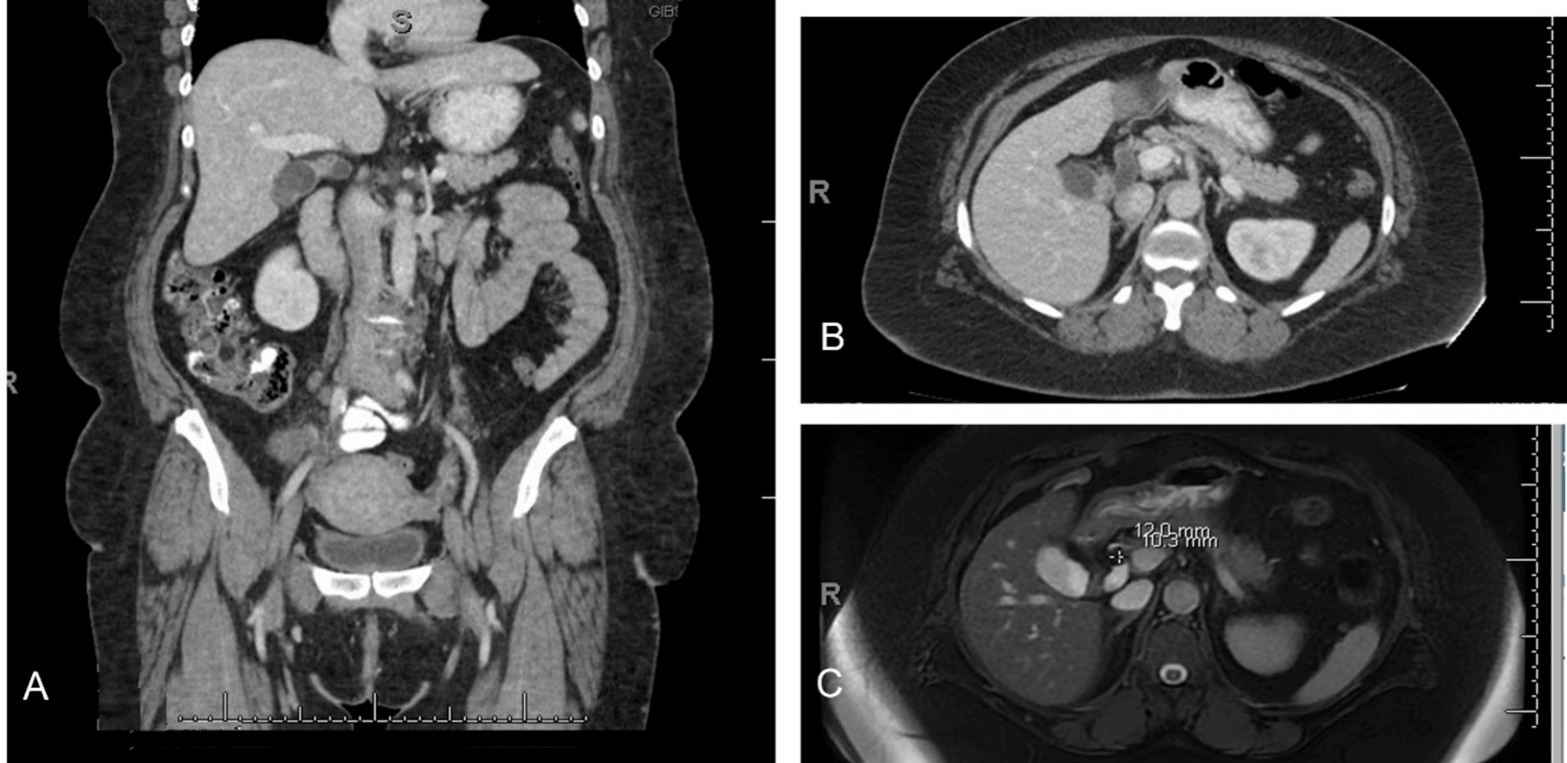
Coronal CT image (A), axial CT image (B), and axial MRI image (C) showing a circumferential, nodular wall thickening of the proximal gallbladder body with associated enhancement and mild diffusion restriction.
